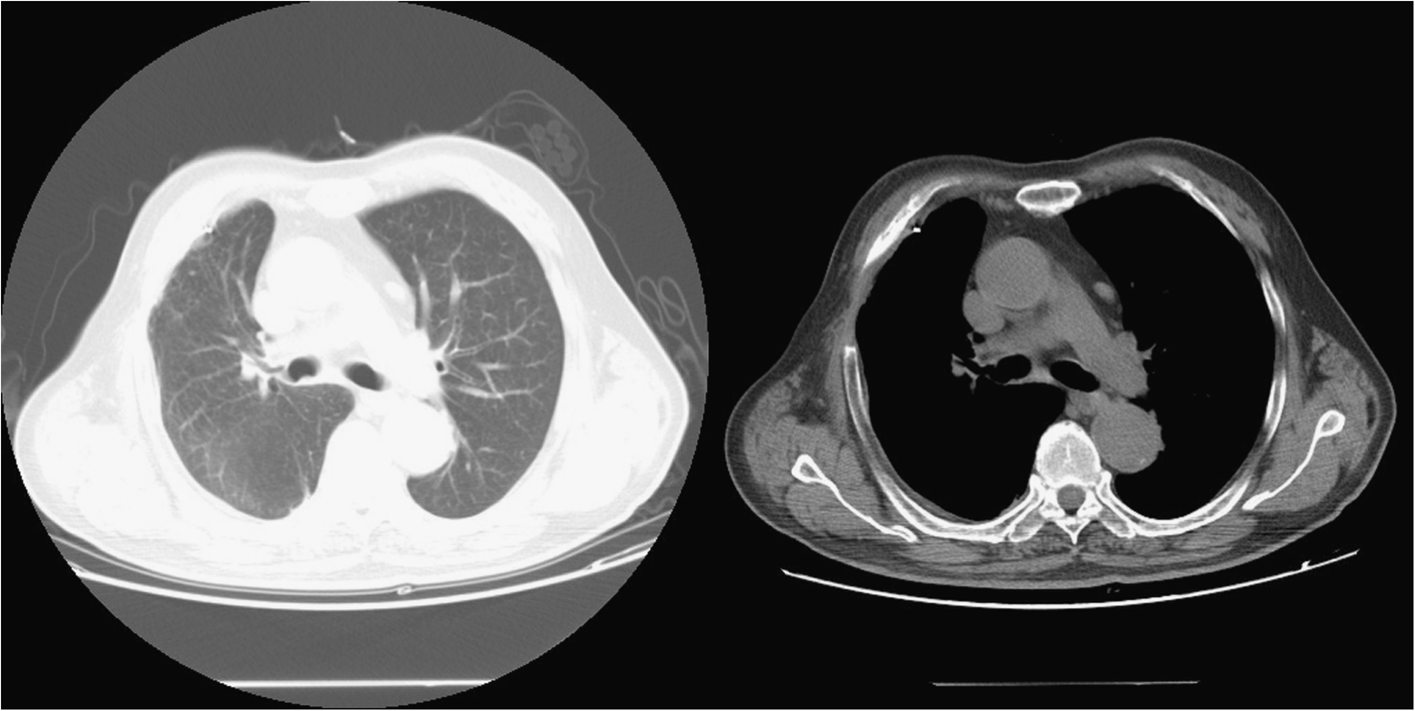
Third chest CT scan on 18 October, 2012.
